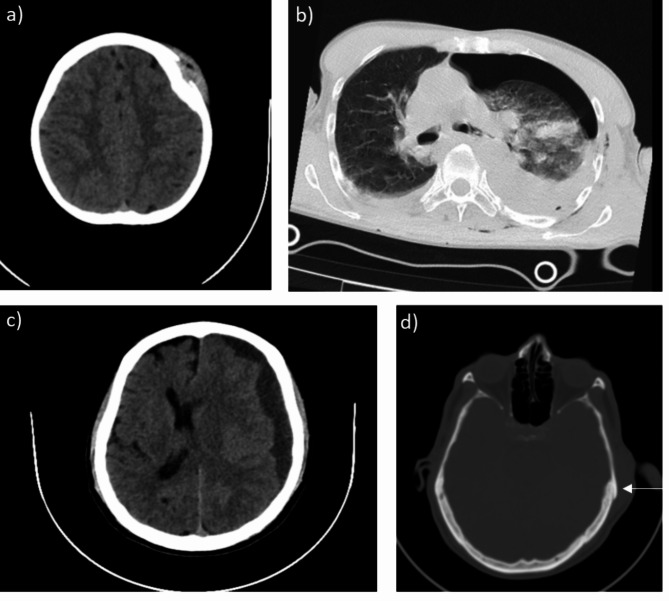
a) plain CT showing depressed frontal skull fracture b) plain CT showing left sided hemopneumothorax c) plain CT showing SDH with midline shift d) Plain CT showing undisplaced left temporal bone fracture (arrow)
